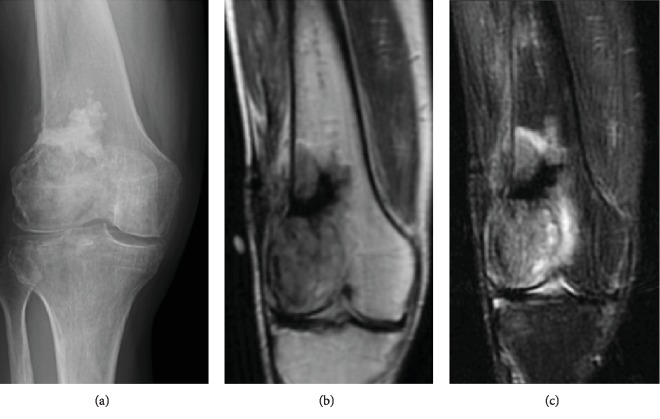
(a) Post-EBRT X-ray of the involved distal femur shows some periosteal reaction developing. Intramedullary sclerotic changes are seen reflecting the introduction of bone cement after the initial biopsy. (b) Postradiotherapy MRI demonstrating the lesion has increased in size since the previous examination now measuring 8.5 cm in the cephalocaudal dimension and 4.5 cm in the transverse dimension with evidence for postoperative change within the lesion. Patchy areas of increased signal within the medullary space of the mid to distal femur as well as the proximal tibia and patella most likely are related to postradiation change. The focal prominent region of decreased signal within the lesion corresponds to interval biopsy, curettage, and cementation. (c) Dark heterogeneous signal on T1W and brighter signal intensity on T2W sequences.
